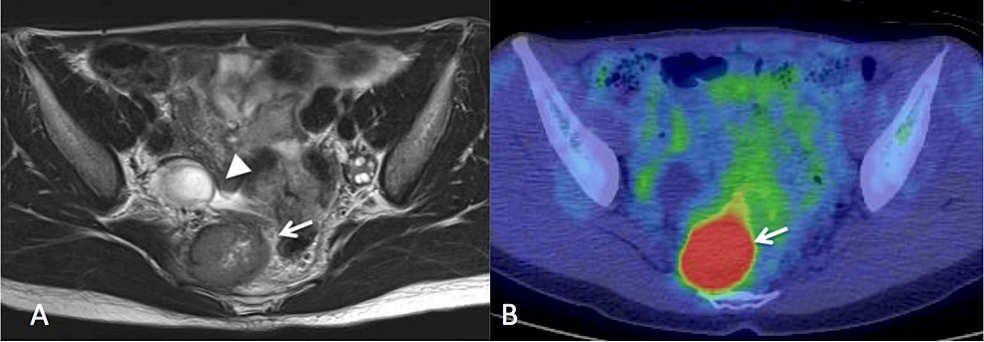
A. Axial view of a T2-weighted magnetic resonance image. Tumor (arrow) and normal right ovary (arrowhead) are observed; B. Positron emission tomoguraphy/computed tomography. An abnormal uptake of [18F]fluoro-deoxy-D-glucose (SUVmax of 20.8) is observed.
